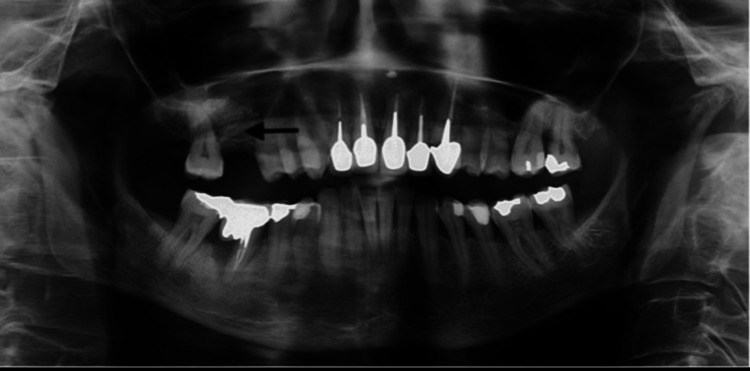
X-ray control after sinus grafting X-ray control after right maxillary lateral approach sinus grafting (β TCP/HA) (arrow). β-TCP: beta-tricalcium phosphate
